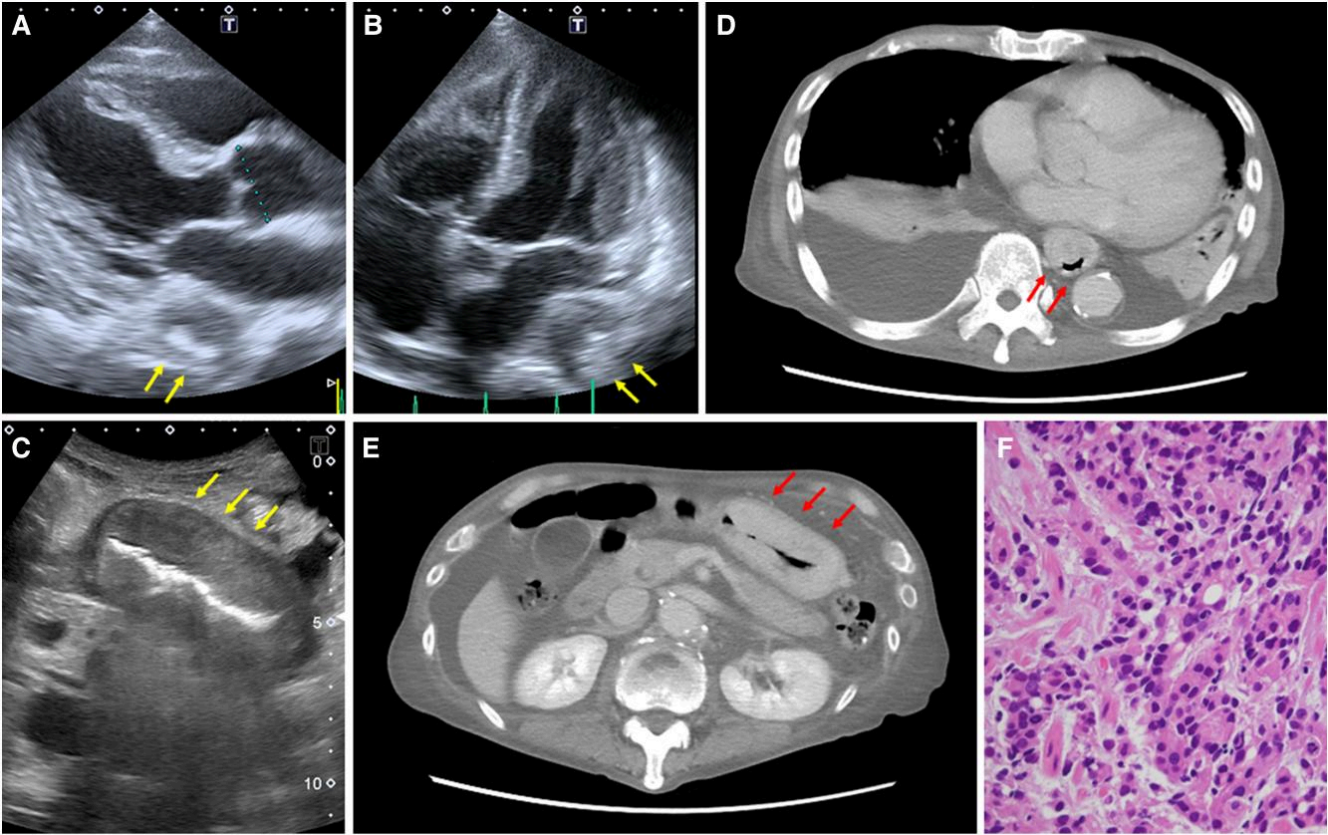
A transthoracic echocardiogram showed an extra-cardiac lesion measuring 33 × 30 mm located immediately adjacent to the left atrium. The lesion was characterized by a large hypoechoic mass with a central hyperechoic area, the so-called pseudo-kidney sign (*A* and *B*, arrows). An abdominal ultrasound also revealed pseudo-kidney sign in the stomach (*C*, arrows). Computed tomography scans showed that these lesions were located in the oesophagus and stomach (*D* and *E*, arrows). The pathological examination showed poorly differentiated gastric adenocarcinoma (*F*).
